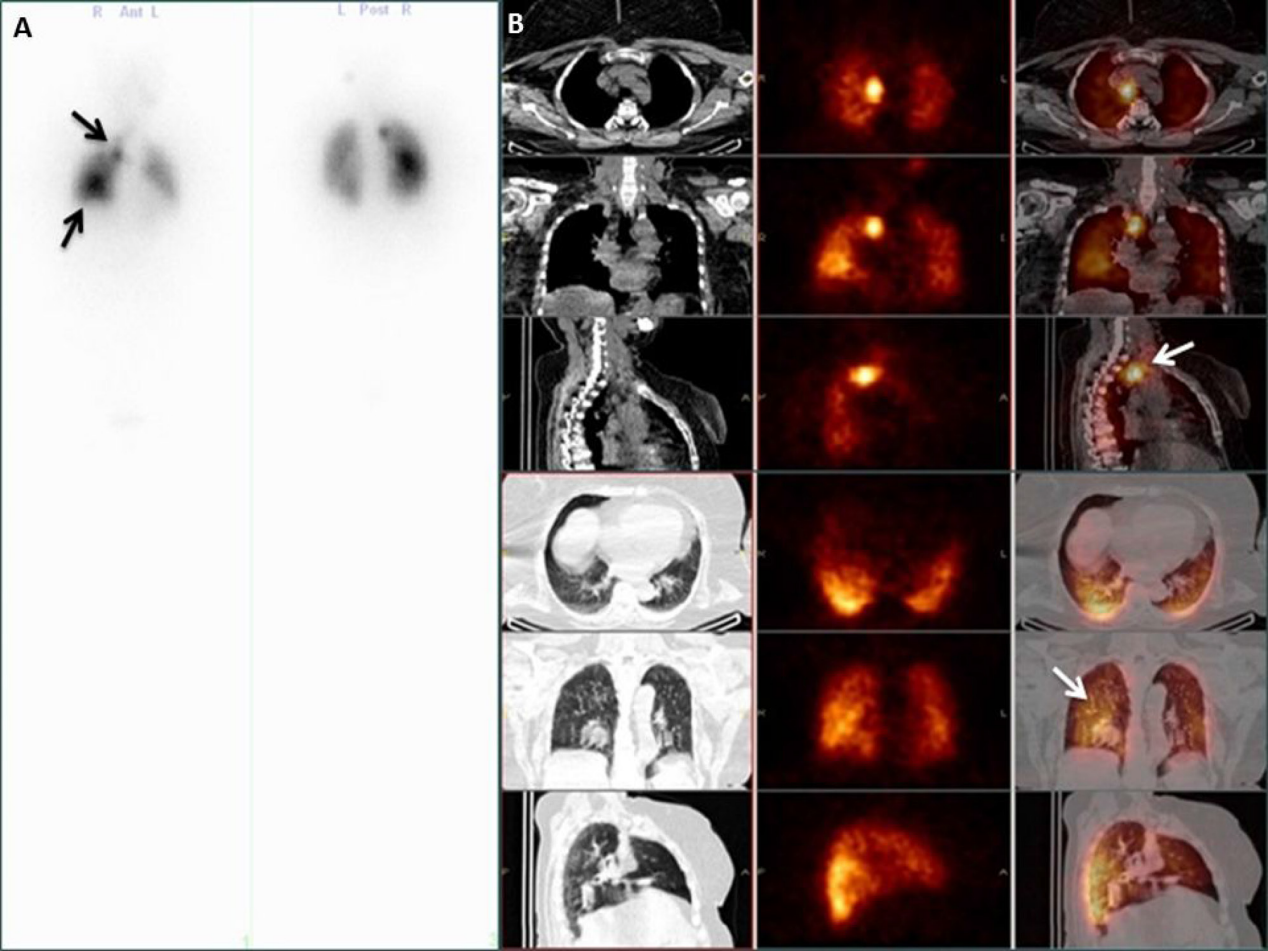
Anterior and posterior whole body scan (A) and SPECT/CT (B) images of a papillary cancer patient with lymph node and pulmonary metastases (arrows).
